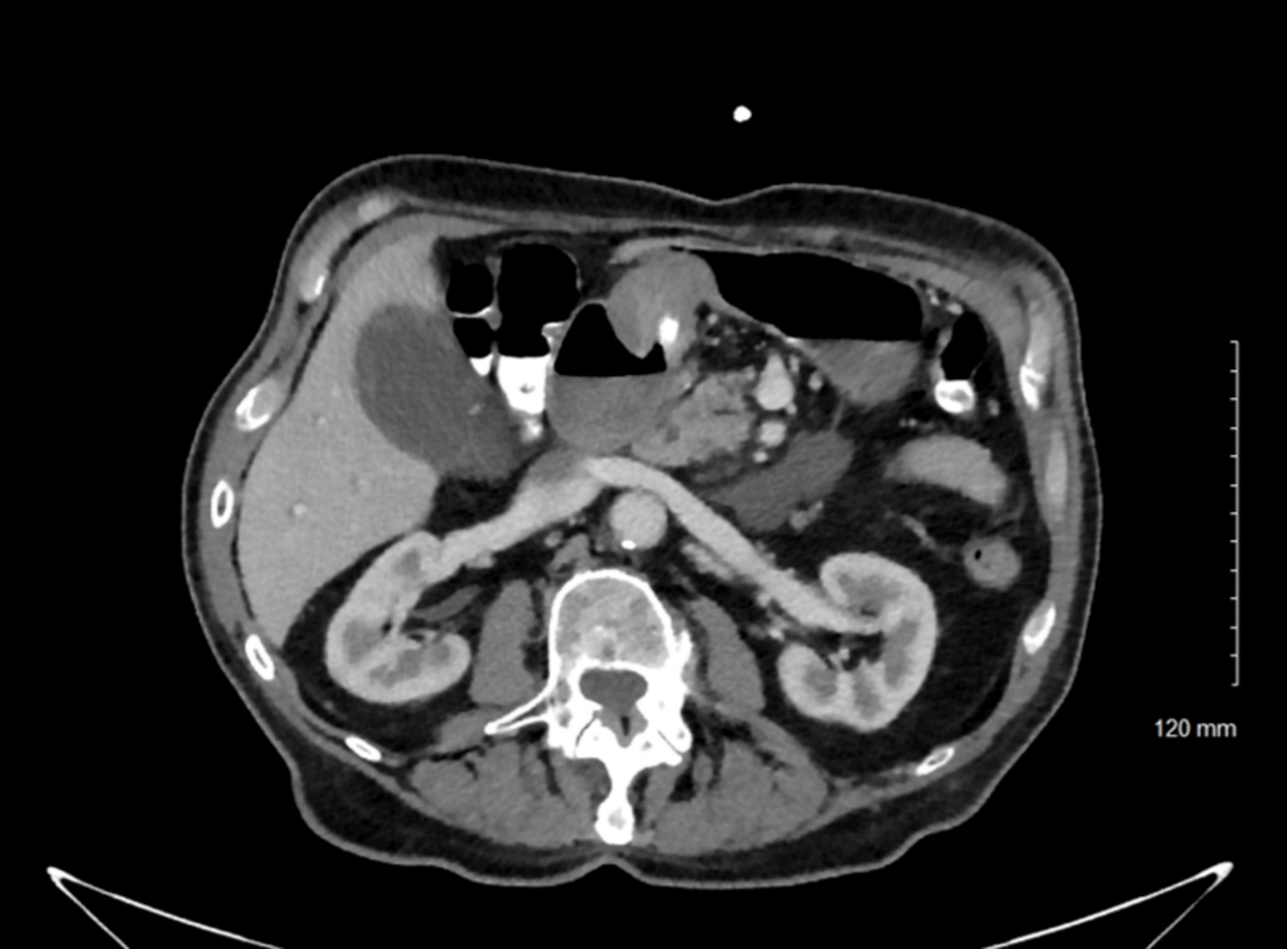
CT abdomen pelvis CT imaging showing pericholecystic and peripancreatic fluid concerning for acute panceatitis
